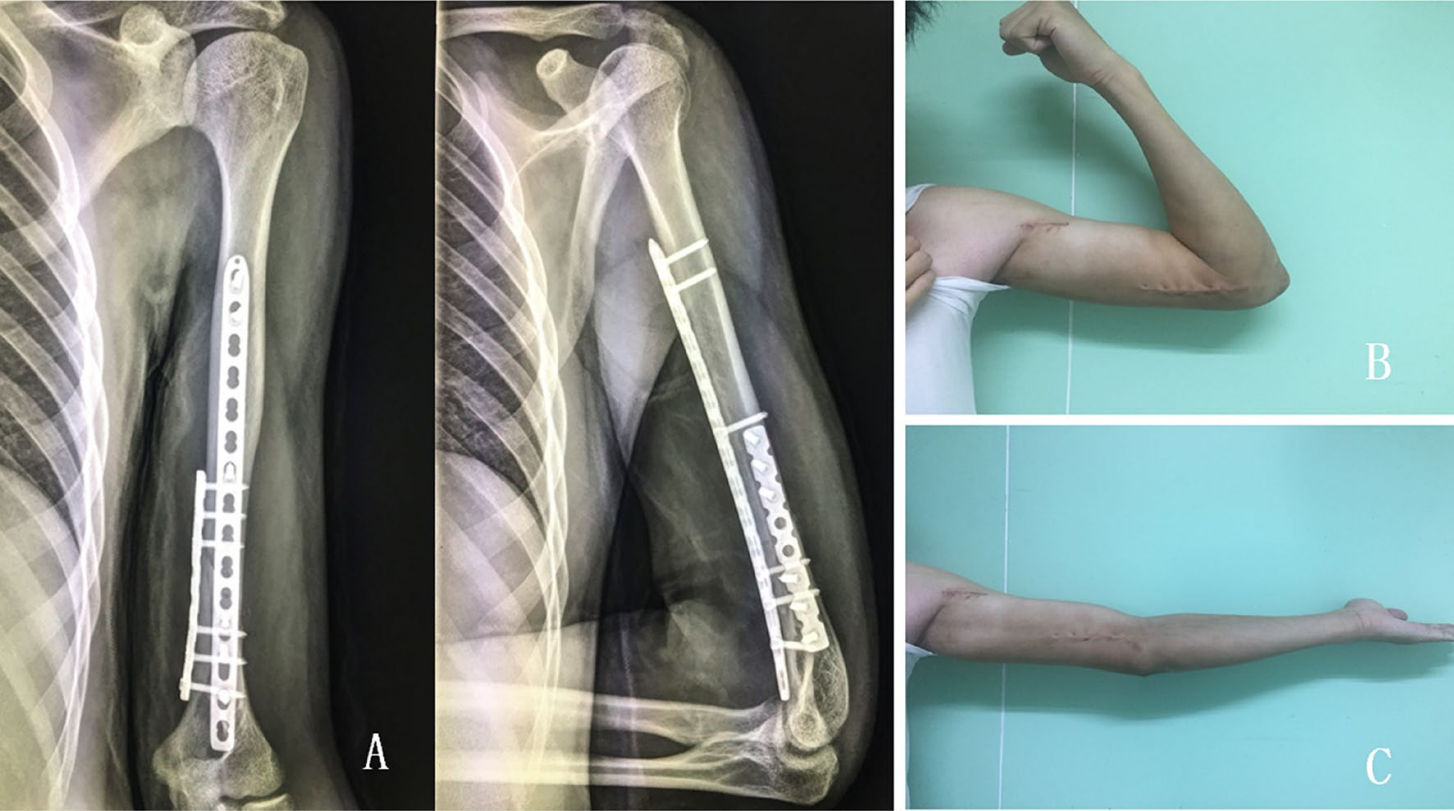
Clinical and radiological outcomes of a patient with distal third diaphyseal fracture of humerus. (A) The final follow-up X-ray; (B,C) the range of motion at the final follow-up of a 34 year-old male was shown.
